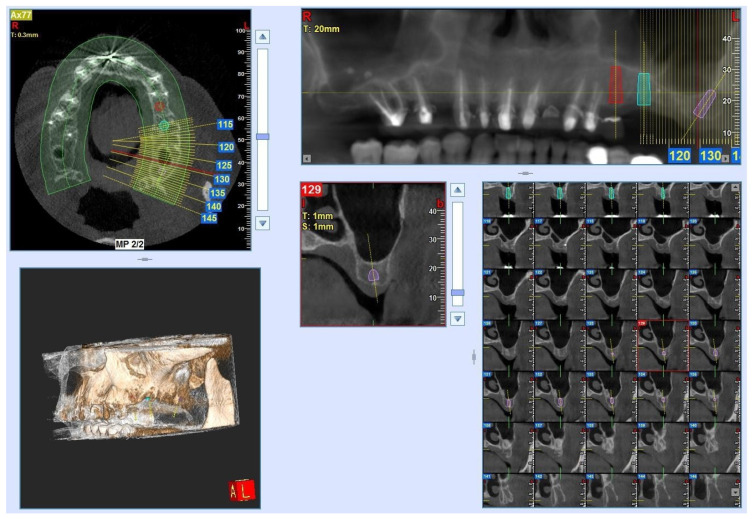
Cone–beam study (CBCT) (FOV 11 × 5 cm^2^). The resolution was 300 µm for planning implant rehabilitation.
